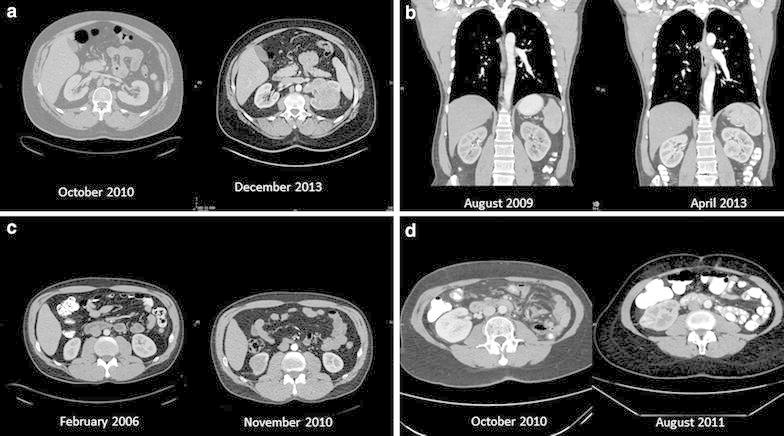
Computerized tomographic scans of selected patients. a A 52-year-old man, on October 2010 normal kidneys were found and on December 2013, a 9 cm clear cell tumor with IVC invasion was found (growth rate 2.84 cm/year). b A 51 year-old-man, on August 2009 normal kidneys were found and on April 2013, 4.5 cm clear cell tumor was found (growth rate 1.23 cm/year). c A 45 year-old man on February 2006 normal kidneys were found and on November 2010, a 4 cm chromophobe carcinoma was found (growth rate 0.84 cm/year). d A 64-year-old lady that had left nephrectomy on May 2009. CT done on October 2010 showed normal right kidney. On August 2011 the patient had a 2.8 cm clear cell carcinoma (growth rate 3.36 cm/year)
